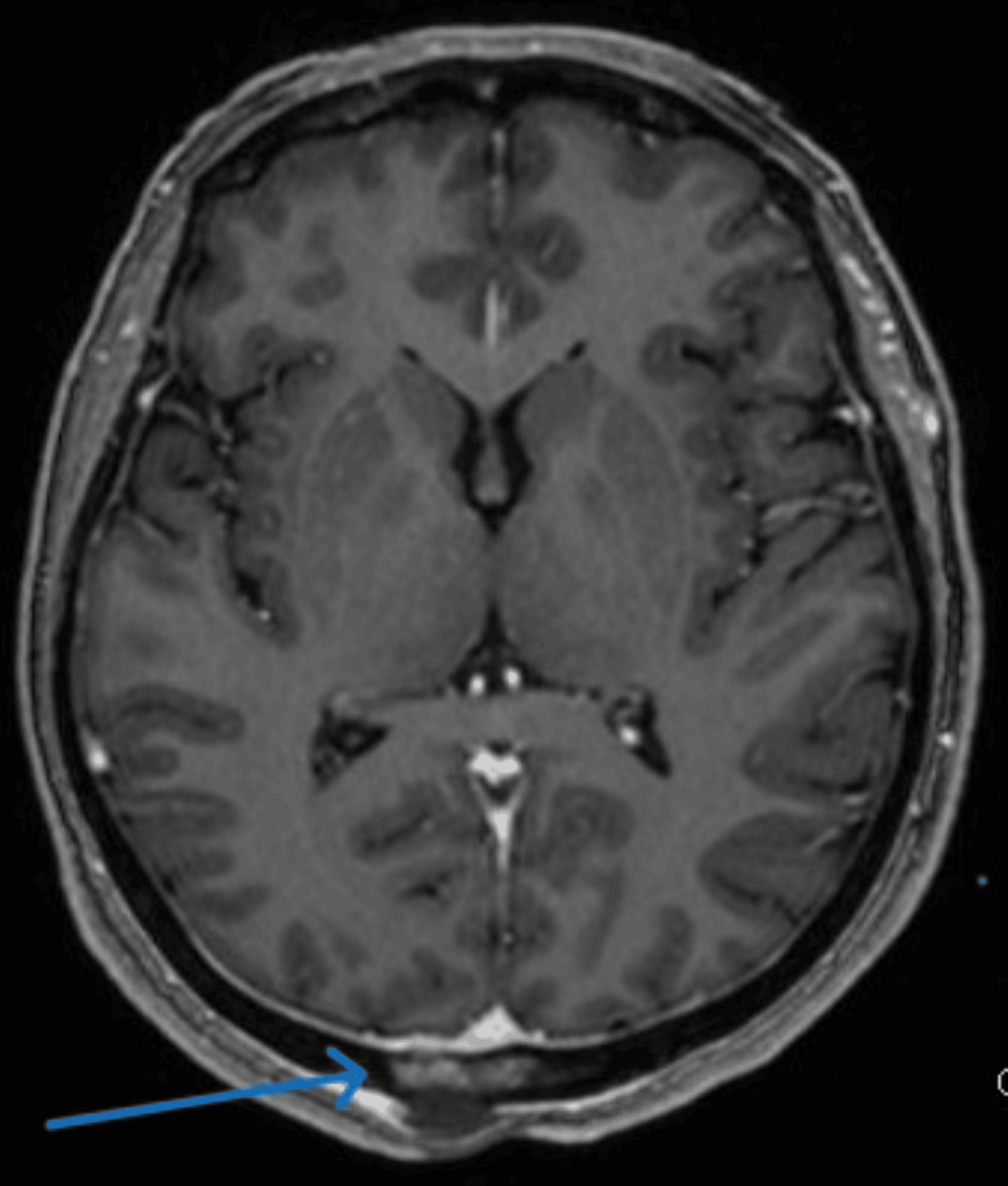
Enhanced axial T1 MRI of the brain demonstrates a right para-midline occipital bone-enhancing lesion with minimal intracranial soft tissue component. No evidence of intraparenchymal lesion or invasion of the venous sinus is noted.
